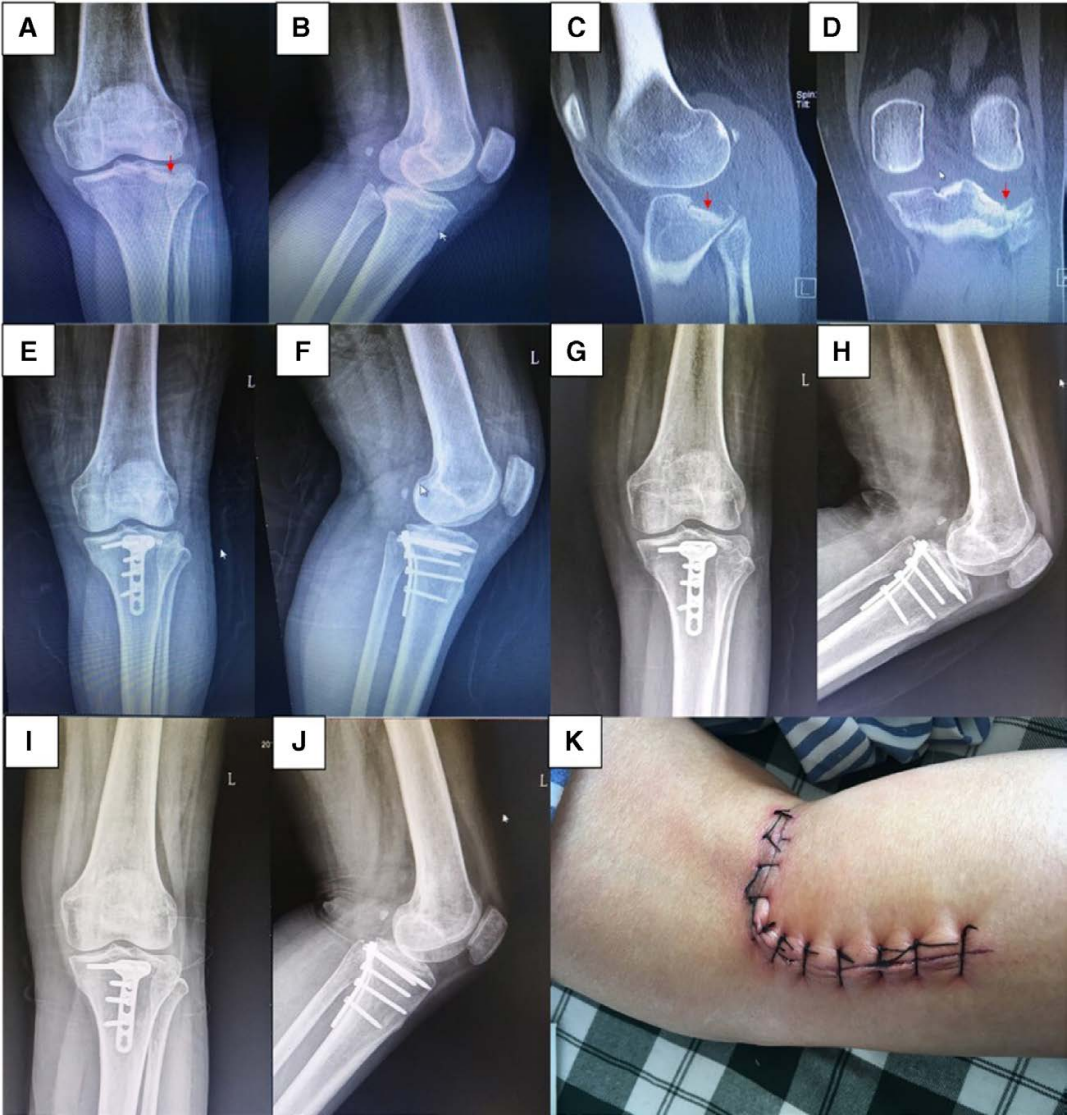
A 55-year-old female suffered from posterolateral tibial plateau fracture caused by traffic accident. X-ray and CT scan (a–d) revealed a Schatzker type III fracture with subsidence of lateral surface (red arrow). Postoperative radiograph 2 days (e, f), 3 months (g, h) and 12 months (i, j) after surgery showed the restoration of lateral joint surface and stable internal fixation. No obvious recollapse occurred during follow-up period. Skin tension was eased by the use of popliteal dermatoglyphics (k).
